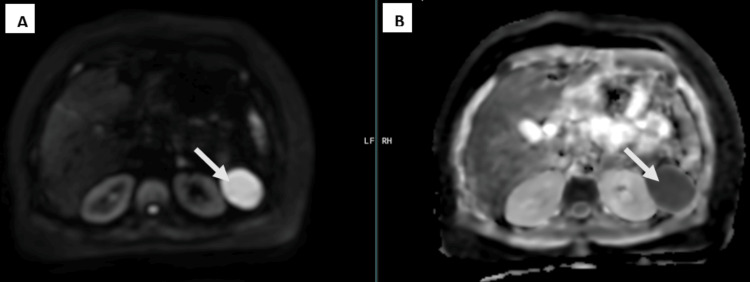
Abdominal MRI (diffusion-weighted imaging) (A) Diffusion-weighted image demonstrating a well-defined lesion (arrow) in the splenic fossa with high signal intensity. (B) Corresponding apparent diffusion coefficient (ADC) map showing low signal, with signal characteristics comparable to splenic tissue.
